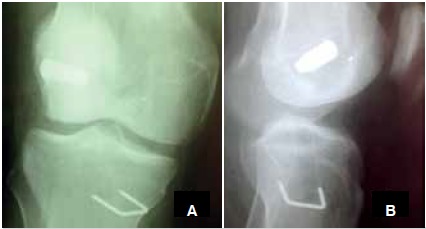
Postoperative X-rays: (A) AP and (B) lateral views showing tibial fixation with staple (right knee).
